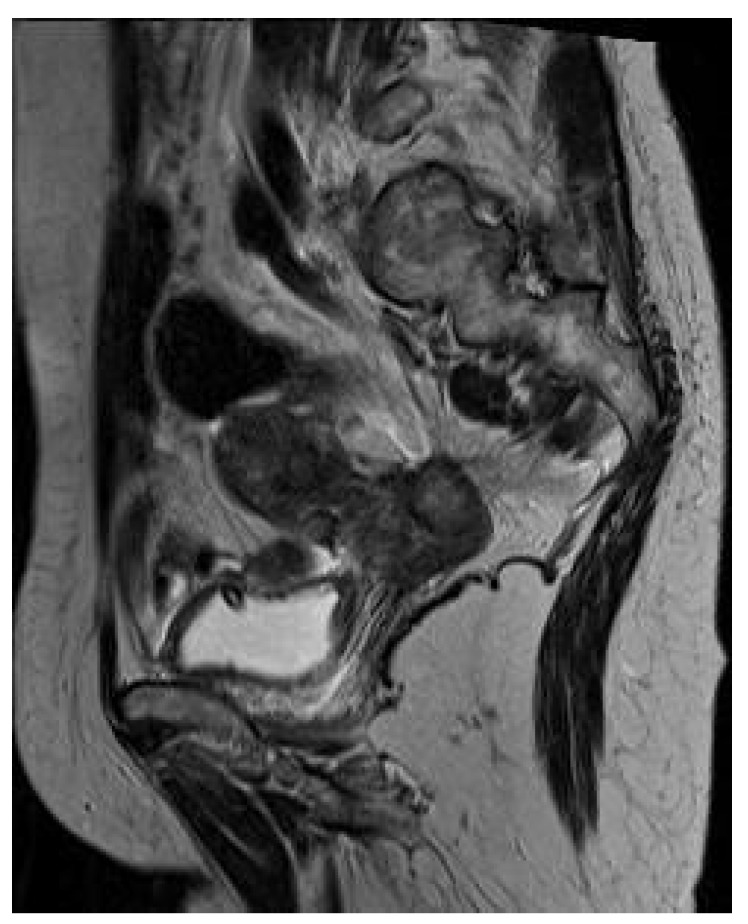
Pelvic MRI revealing the presence of a large tumoral nodule compressing the right ureter.
